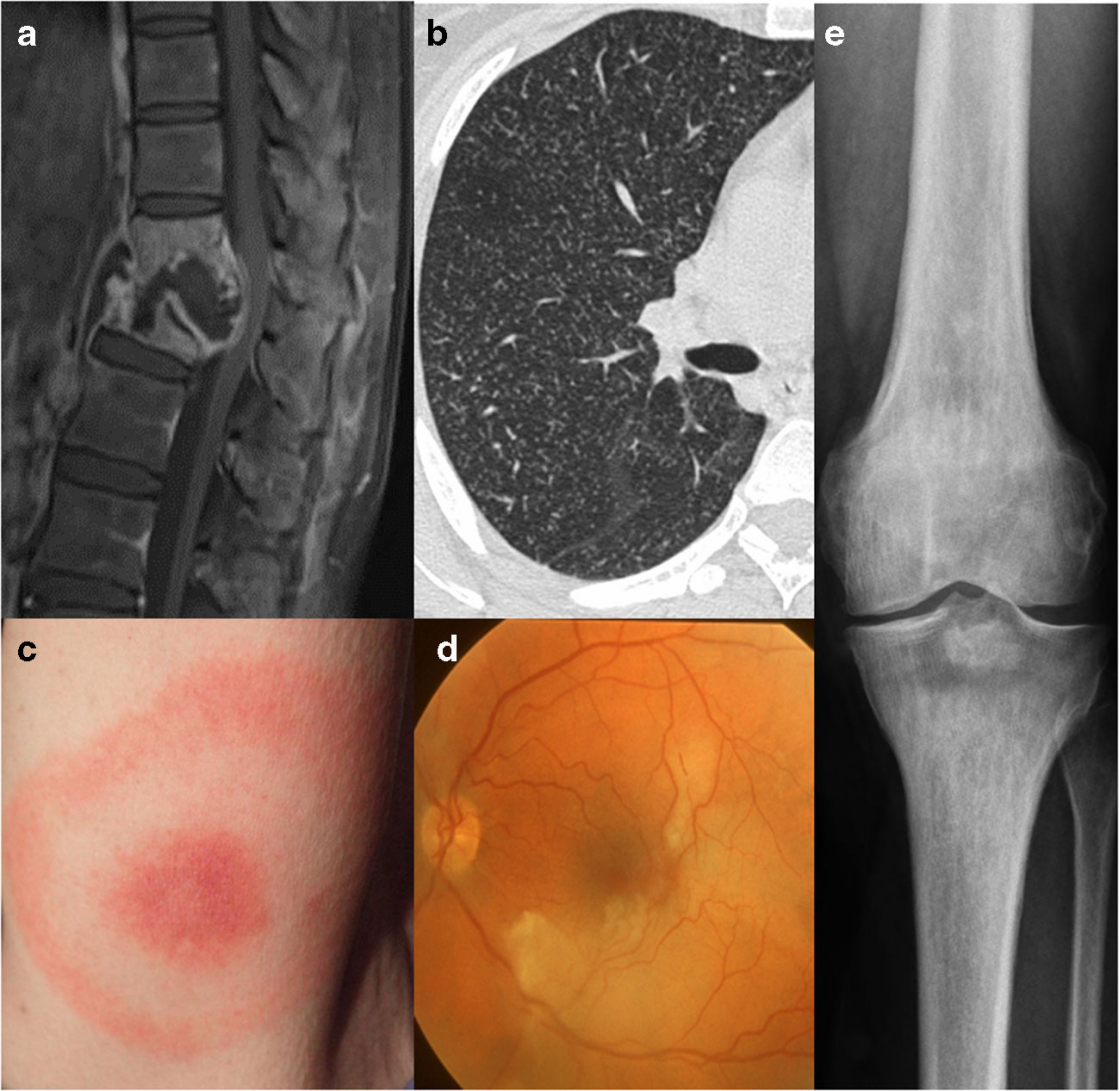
Systemic involvement. a A 26-year-old female with neurotuberculosis. Sagittal post-contrast T1-weighted MRI with fat saturation shows collapse of thoracic vertebrae, destruction of the interposed vertebral disc, prevertebral and intervertebral fluid collection with peripheral enhancing margins and diffusely enhancing remainder of the thoracic vertebrae, suggesting spondylodiscitis with abscess formation, known as Pott’s disease. Due to displacement and mass effect narrowing of the spinal canal with compression of the spinal cord. b Axial high-resolution CT scan (right lung) in the same patient showing ‘miliary tuberculosis’ with innumerable small pulmonary nodules with centrilobular distribution. c A 37-year-old female with a typical erythema migrans, as a disease-specific finding in Lyme disease. d A 20-year-old female affected by Susac’s syndrome with branch retinal artery occlusion. Fundus photography of the left eye shows ischaemic retinal oedema and narrowed branches of the superior and inferior temporal arteries. e A 24-year-old female affected by Erdheim–Chester disease with a sclerotic metaphyseal lesion in the tibia as a disease-specific finding
